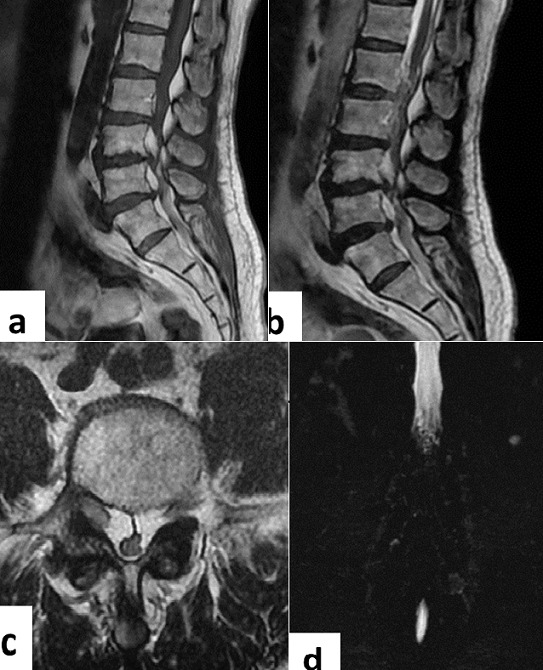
(A) sagittal T1 weighted images MRI shows posterior and anterior epidural adipose tissue compressing the dural sac extending from L1 to L5 segment with high signal intensity; (B) sagittal T2 weighted images MRI featured multilevel high intensity epidural mass lesion compressing the dural sac; (C) axial T2 weighted images MRI noticed a hyperintense anterior epidural mass with deformation of the dural sac; (D) frontal MRI myelography shows complete stop of the cerebro-spinal fluid on the lumbar spine
